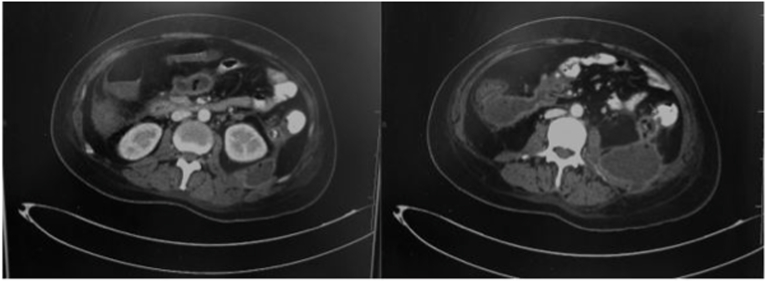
A+2B: The abdominal CT scan showed an extensive necrosis with the presence of air bubbles, and the existence of a fistula of the descending colon in the left retroperitoneal space.
